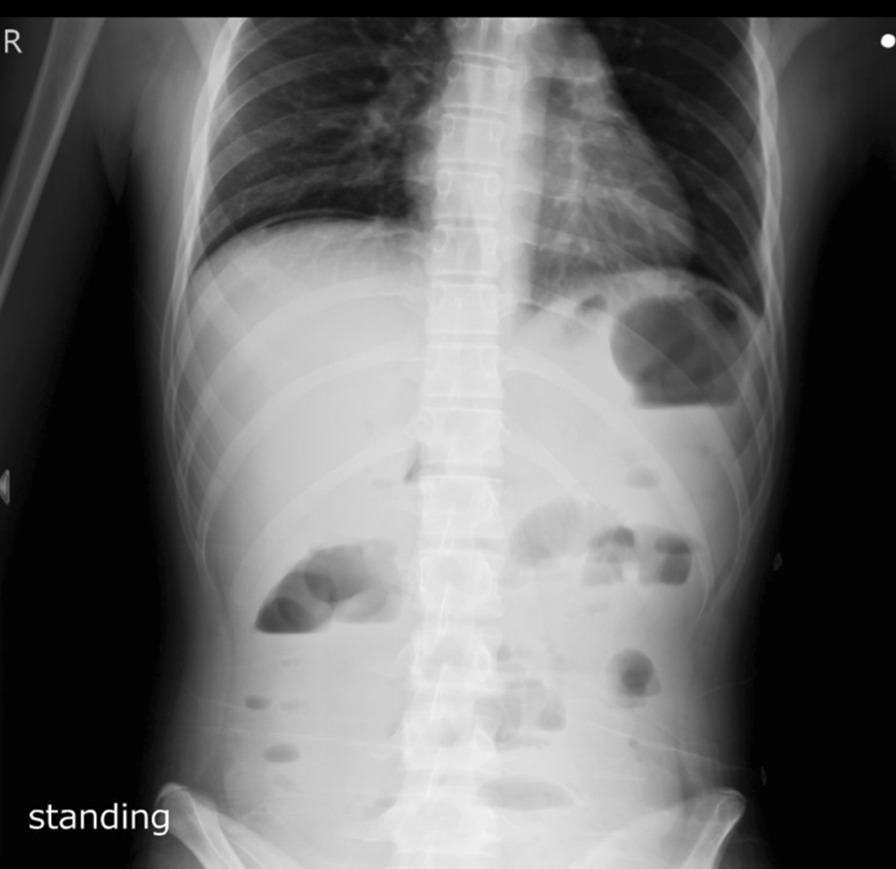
The standing view of abdominal plain film showed subdiaphragmatic free air
